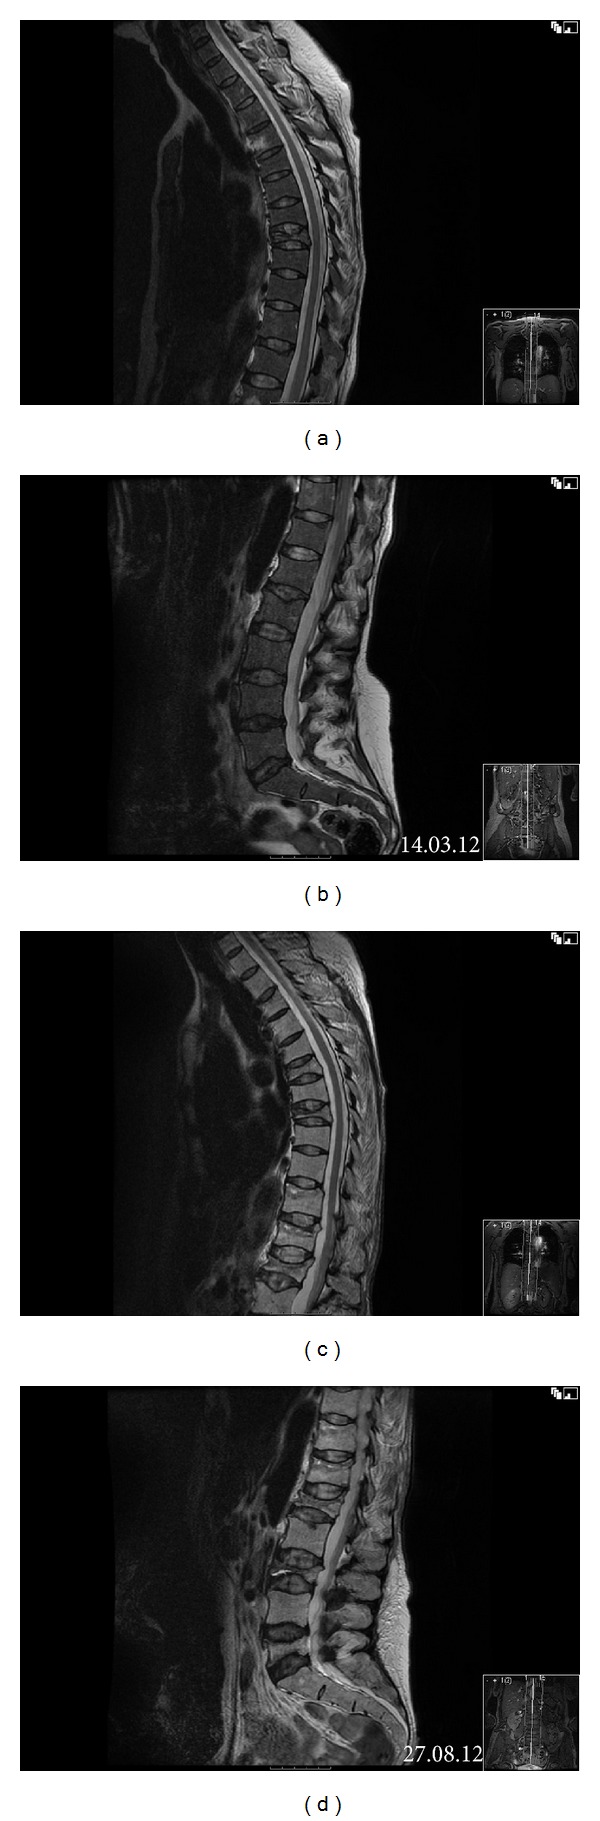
MRI spine. T2 fat-saturated axial view at the initial staging showed a compression fracture of T8 (a) and no other osseous lesions (a, b). Five months later, appearances were suspicious for lymphomatous infiltration with abnormal signal in T11 and T12 vertebral bodies and multilevel compression fractures of thoracic spine (c) and lumbar spine (d).
